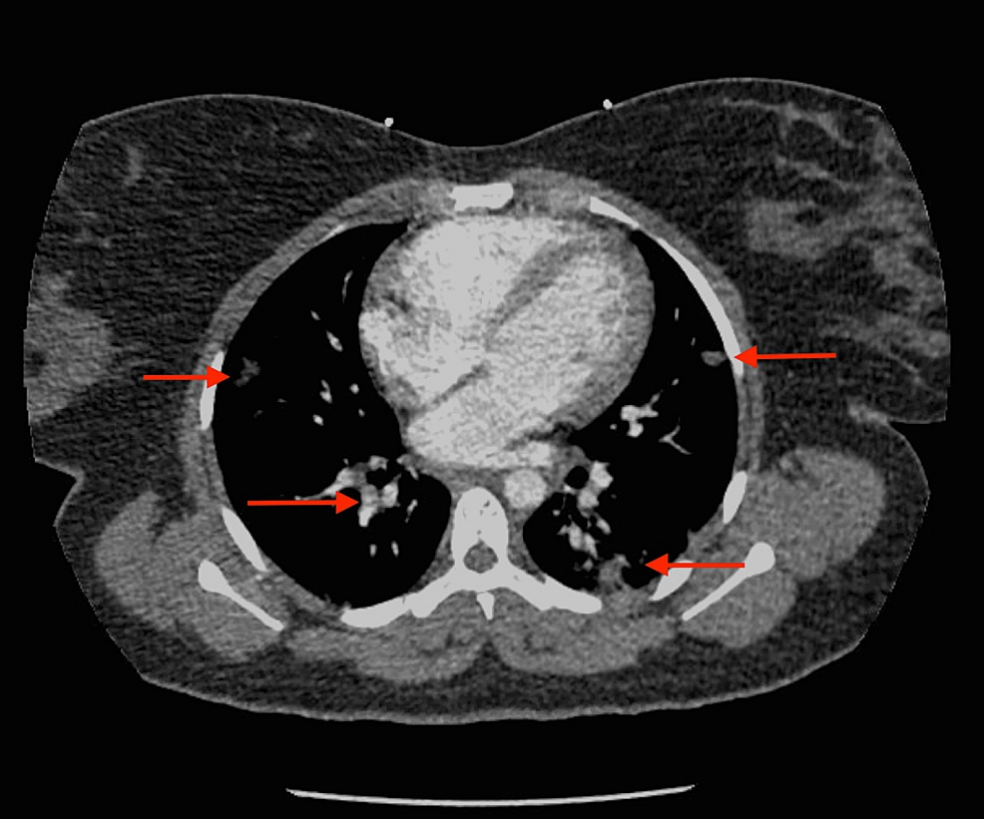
CT scan of the chest with arrows showing multiple bilateral pulmonary masses of varying sizes.
